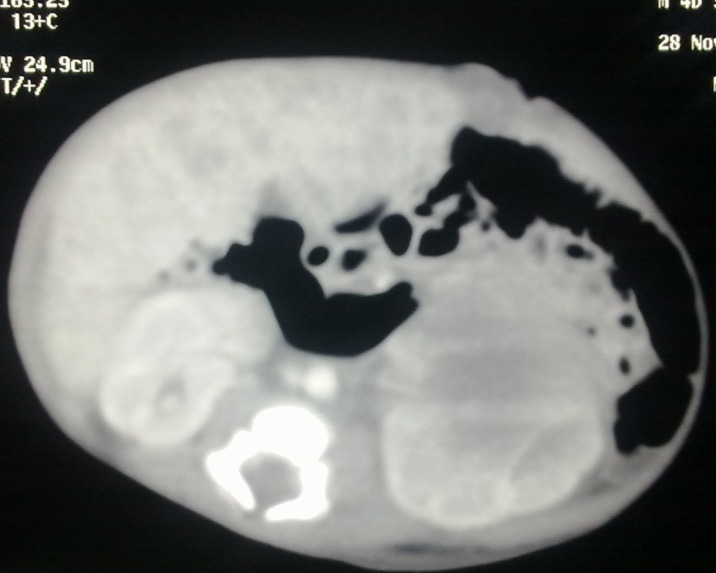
Figure 1:CT scan of abdomen showing heterogeneous masses in both suprarenal (adrenal glands), larger on left side showing solid cystic areas and calcifications, measuring 60 mm x 48 mm. Right side lesion measured 31 mm x 23 mm.
